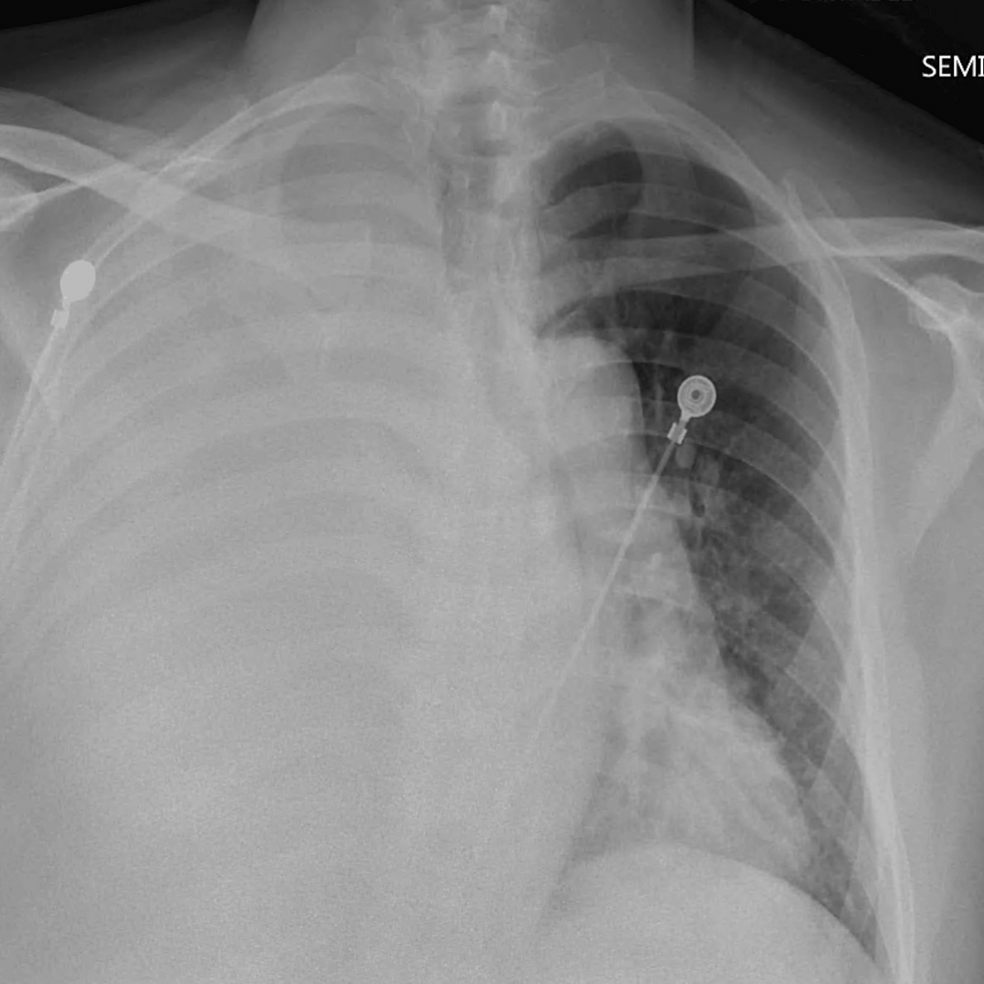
Chest x-ray showed a large right pleural effusion.
